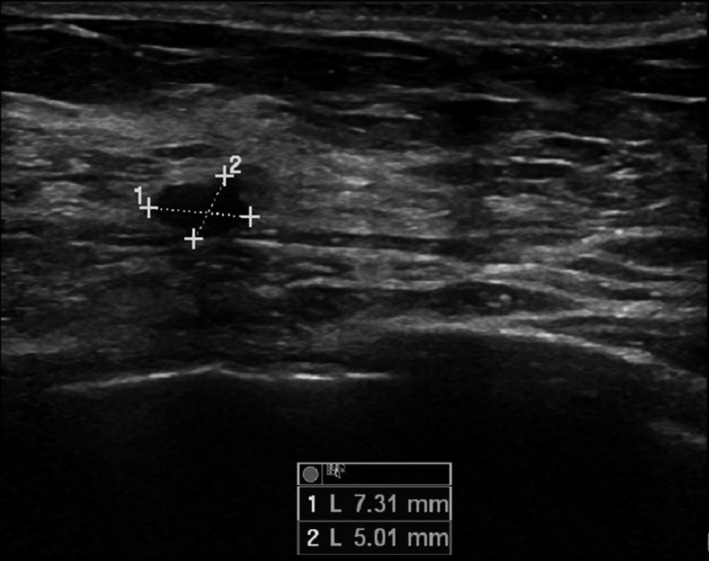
Ultrasound, coronal cut, showing the 5 × 7 mm lumen of the fistulous track.
